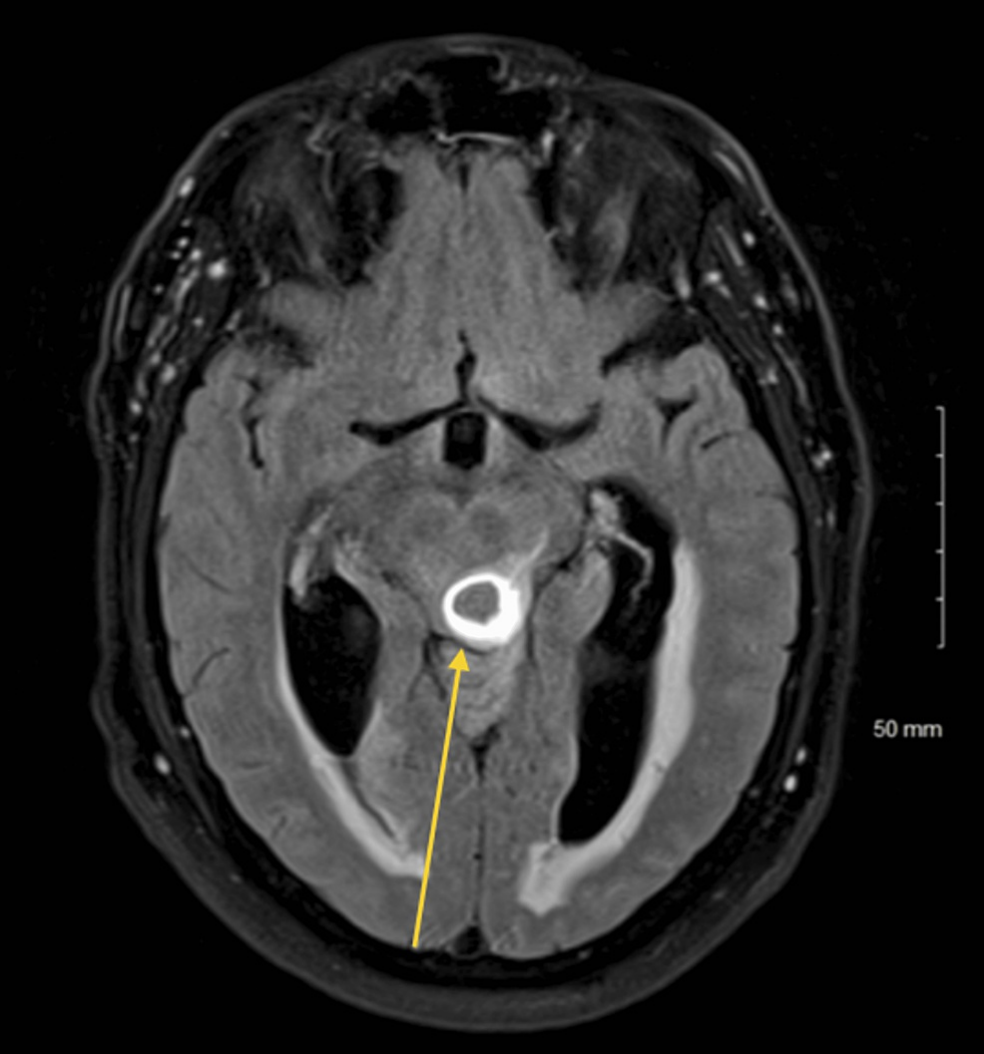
Preoperative MRI Preoperative MRI concerning for multicentric glioma with a dominant lesion involving the brainstem and extending to the left middle cerebellar peduncle greater than the right cerebellar hemisphere and possibly the upper cervical cord.
